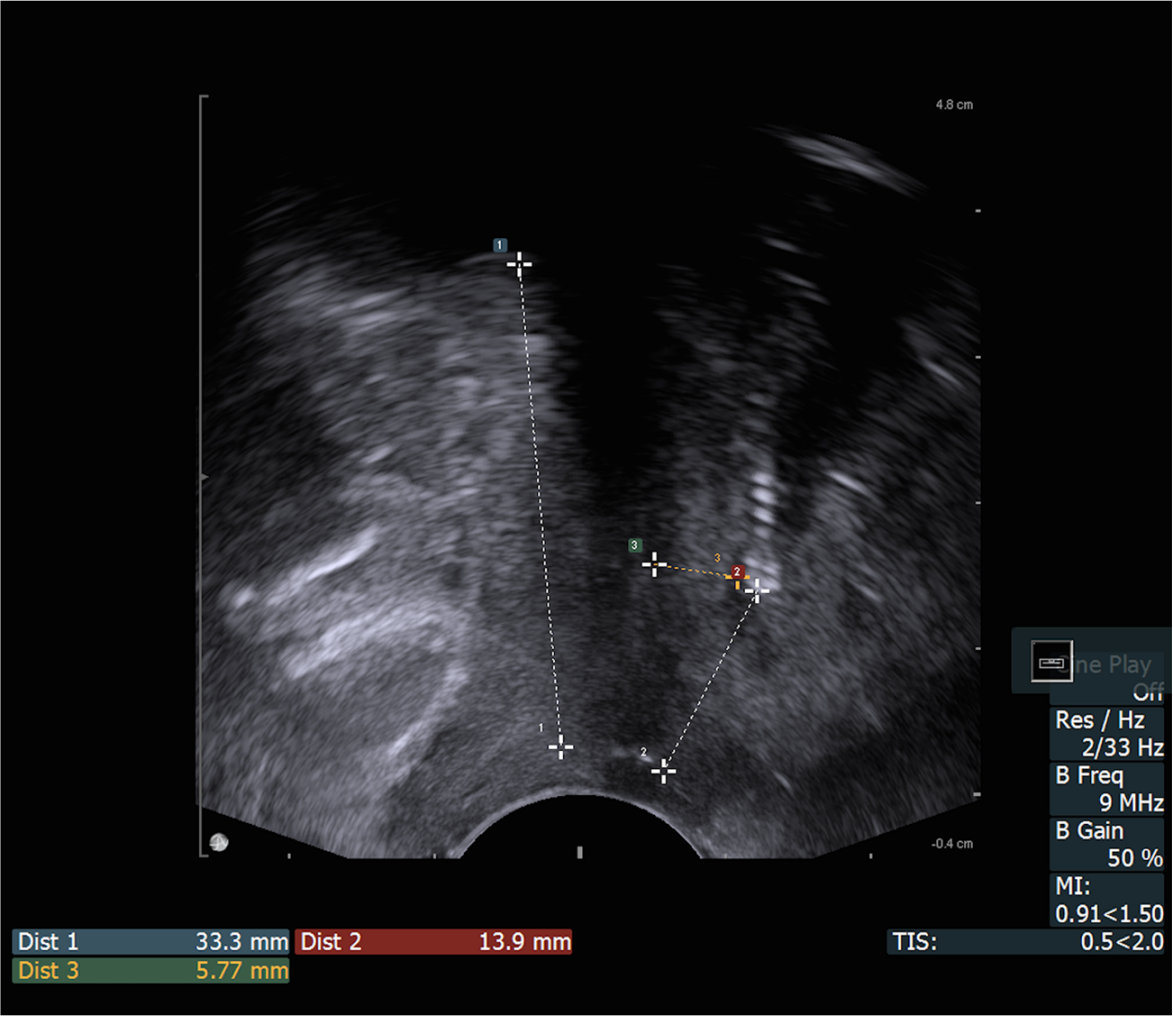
Ultrasound tape visualization in the medio-sagittal plane. *Dist 1*: urethral length. *Dist 2*: distance from the external urethral orifice to the lower edge of the tape. *Dist 3*: distance between the lower edge of the tape and the hypoechogenic urethral complex
